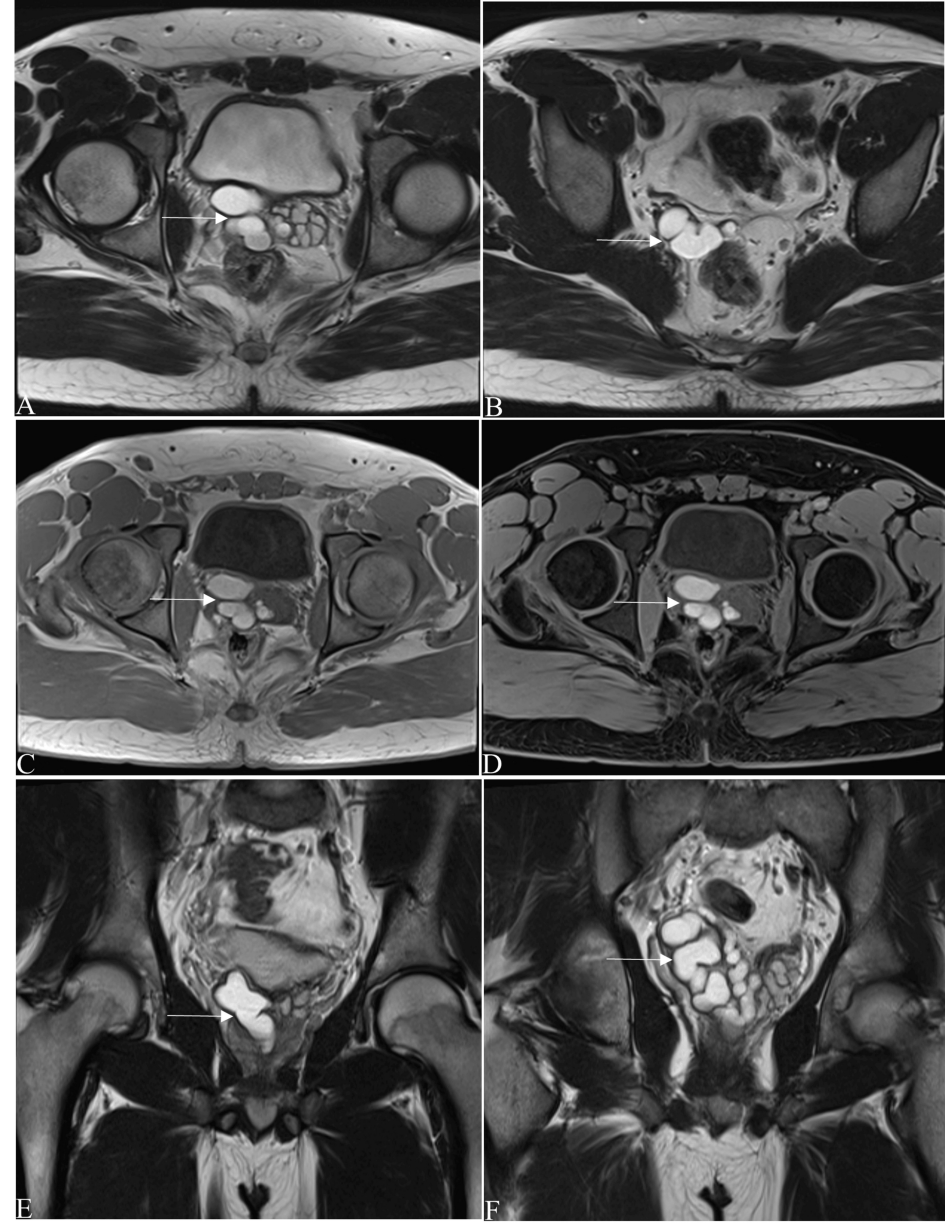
MRI of the pelvis. (A, B) Axial T2W images, (C, D) axial T1W images, and (E, F) coronal T2W images showed a well-defined oblong T1- and T2-hyperintense lesion (white arrows) involving the right seminal vesicle with mass effect on the adjacent structures. T1W: T1-weighted; T2W: T2-weighted.
